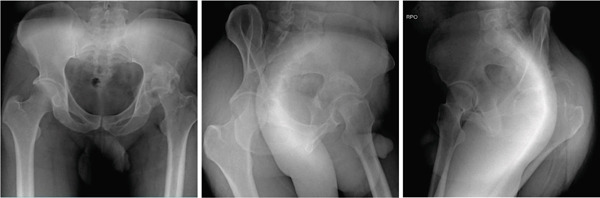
Case 2 preoperative radiographs.
